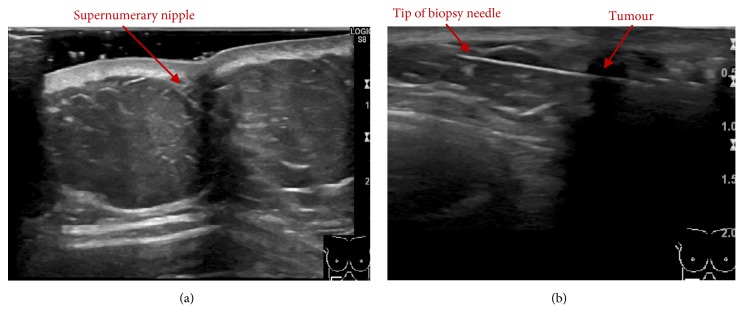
Ultrasound of upper abdominal wall right side (a). Ultrasound guided needle core biopsy (16G) of the tumour located on the upper abdominal wall right side (b).
